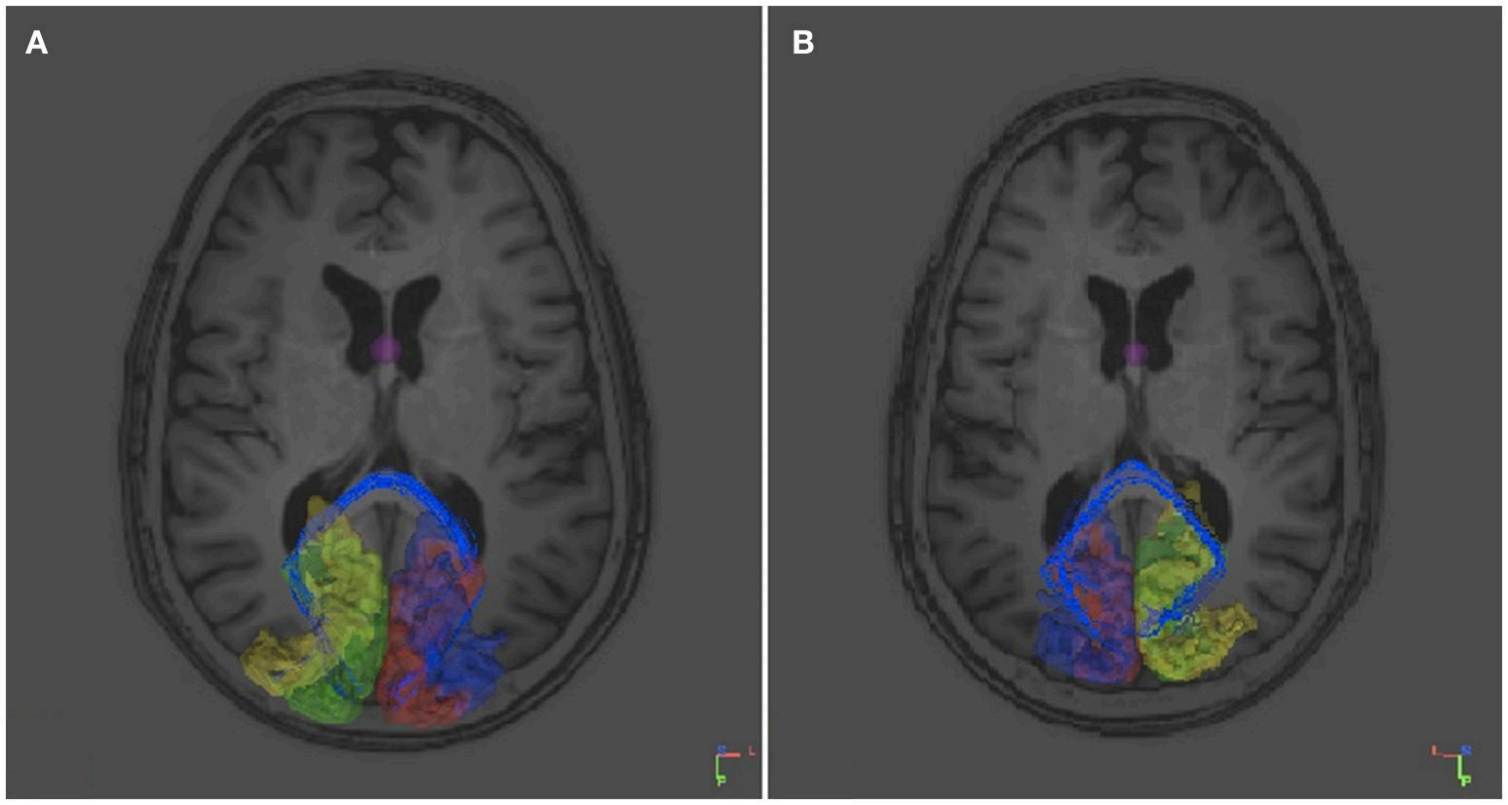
DTI tracts connecting the right and left V1 and V2 in a control subject (RTM 30). In the left occipital cortex V1 is shown in red and V2 in blue. In the right occipital cortex V1 is green and V2 yellow. DTI tracts connecting both visual areas via the corpus callosum are shown in blue. There were no tracts that course through the anterior commissure. (A) An axial plane MRI image is shown from below. Tracts seem to aim for the border area between V1 and V2. (B) An axial plane image shows the same tracts from above.
